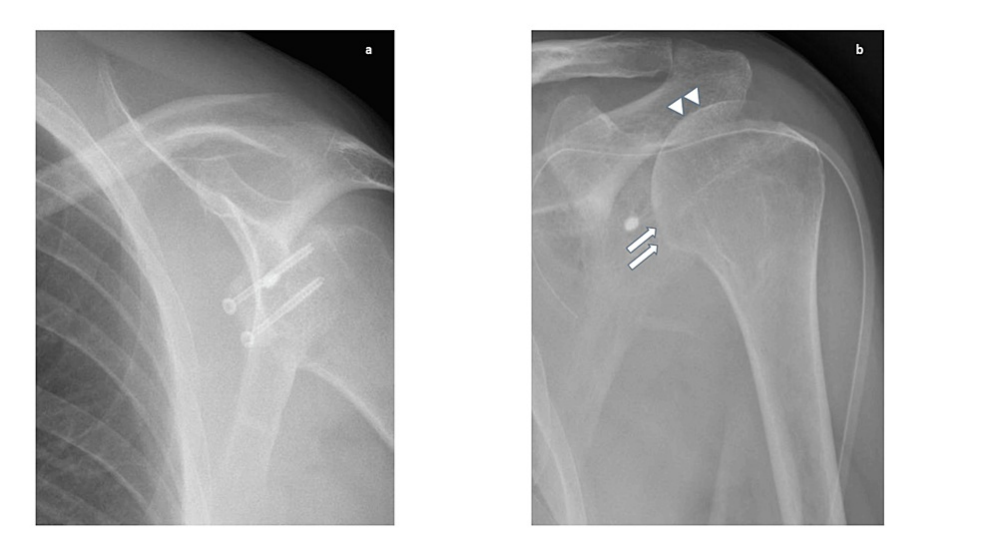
Anteroposterior shoulder radiograph before (a) and after (b) the removal of the compression screws. (b) Humeral head erosion (arrows) and thickening of the cortical bone (arrowheads).
